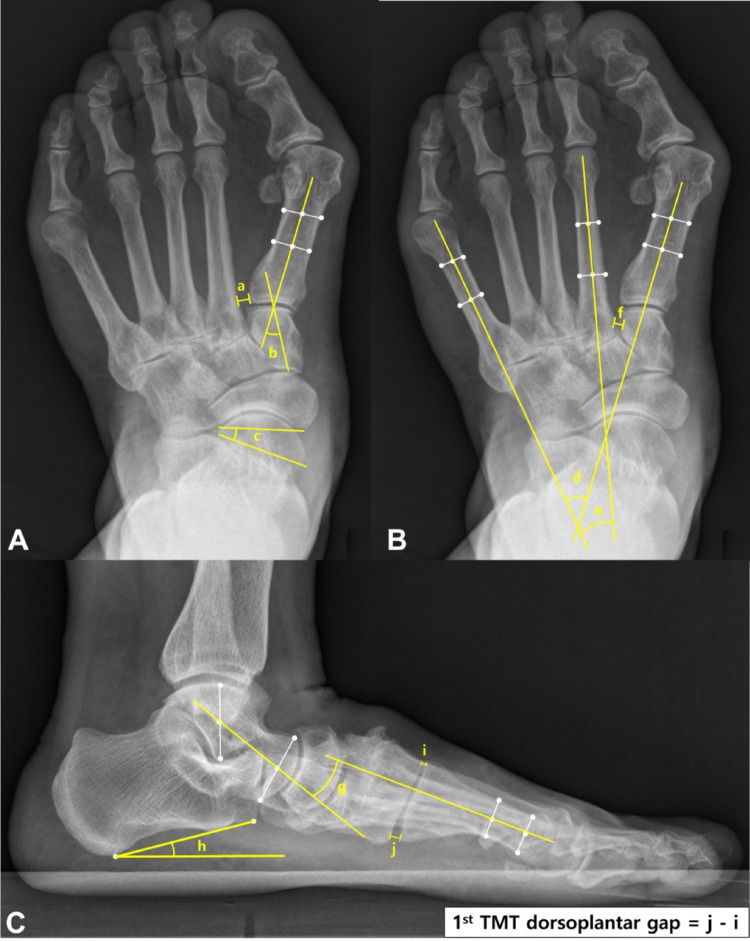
Radiographic measurements on weight-bearing foot radiograph A. First-second metatarsal distance (a), first metatarsal–medial cuneiform angle (b), talonavicular coverage angle (c) B. First-fifth intermetatarsal angle (d), second-fifth intermetatarsal angle (e), medial cuneiform to second metatarsal base distance (f) C. Lateral Meary’s angle (g), calcaneal pitch angle (h), 1 st TMT dorsoplantar gap (j–i). (*TMT* tarsometatarsal)
